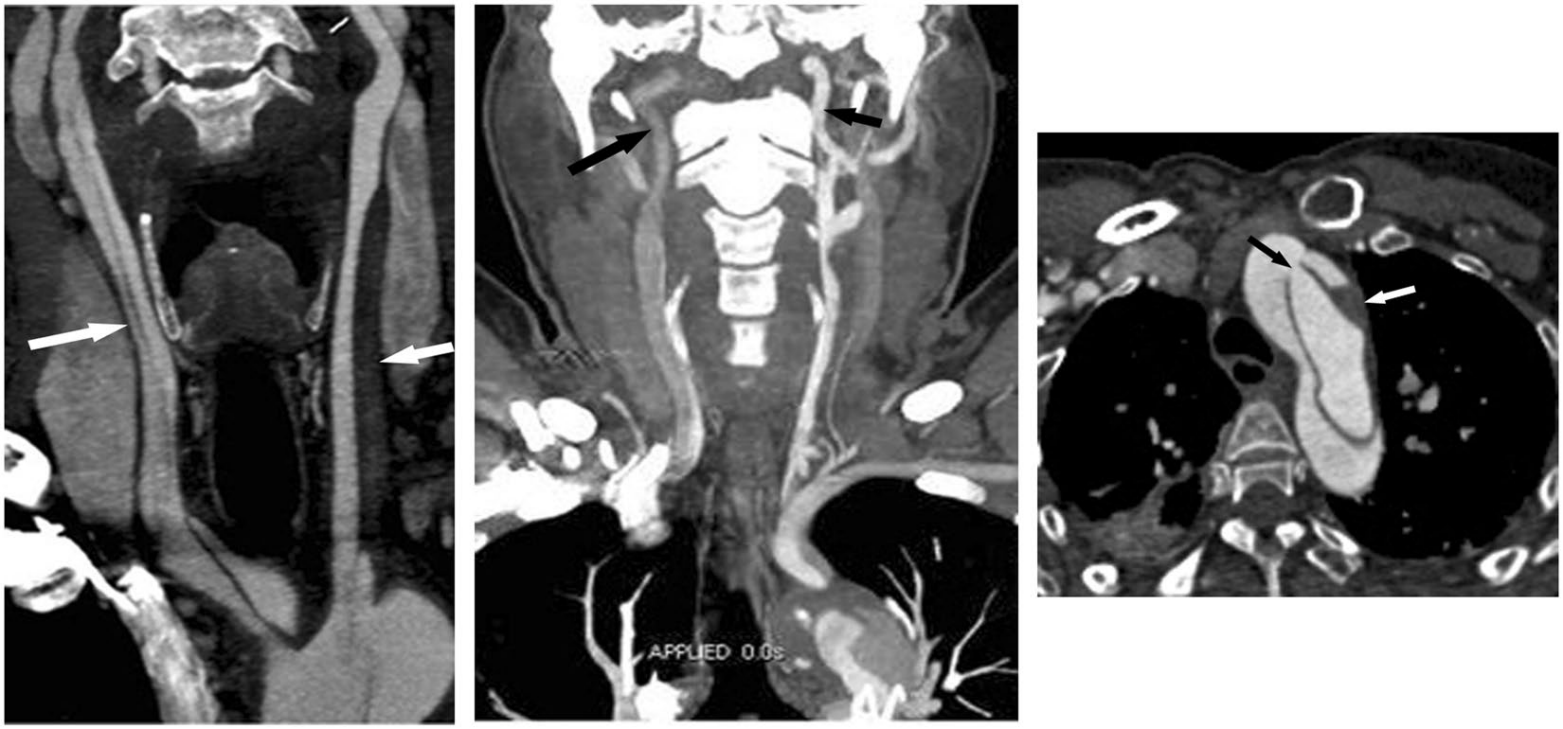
Three CTA Risk Findings for Postoperative TND. (a) Carotid and aortic CTA in a 39-year-old man with ATAAD. Coronal thin MIP image showing aortic dissection extending to both the right (long arrow) and left CCA (short arrow). This finding suggests that dissection involving CCA is an important risk predictor for postoperative TND. (b) Carotid and aortic CTA in a 54-year-old man with ATAAD. Coronal thin MIP image showing that the contrast enhancement of the right ICA (long arrow) is lower than that of the left ICA (short arrow), due to the origin from the false lumen of the right CCA dissection. The results show an inadequate blood supply in the unilateral carotid artery and imply ipsilateral cerebral hypoperfusion. (c) Carotid and aortic CTA in a 49-year-old man with ATAAD. Axial image showing an entry tear located in the aortic arch (black arrow). Note the low density of thrombosis (white arrow) in the false lumen. An aortic arch tear implies an increased risk of pre- and intraoperative embolism into the cerebral artery via the entry point. CTA = CT angiography; TND = temporary neurological dysfunction; ATAAD = Acute type A aortic dissection; MIP = maximum intensity projection; ICA = internal carotid artery; MPR = multiplanar reformation. Note the low-density haematoma in the false lumen of the involved left CCA.
